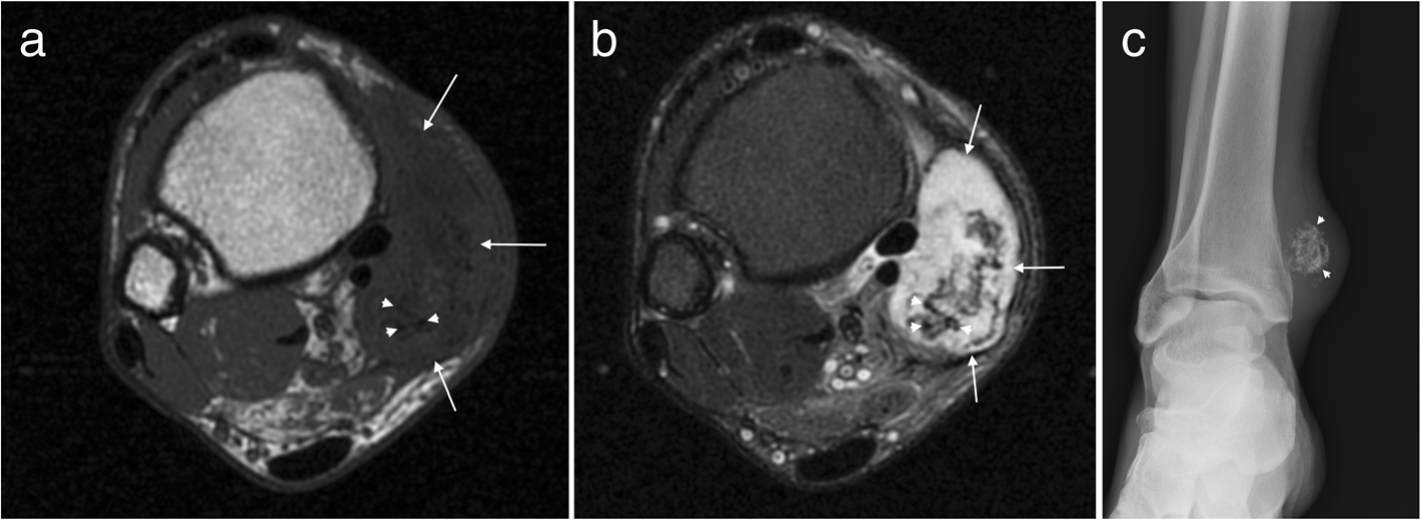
Soft tissue chondroma. Axial T1W (a) and T2FS (b) images of the ankle with a large well-defined posteromedial mass (arrows) which appears T1 hypointense and T2 hyperintense with internal low signal chondroid foci (arrowheads). The chondroid matrix is readily appreciated on the corresponding radiograph (c)
